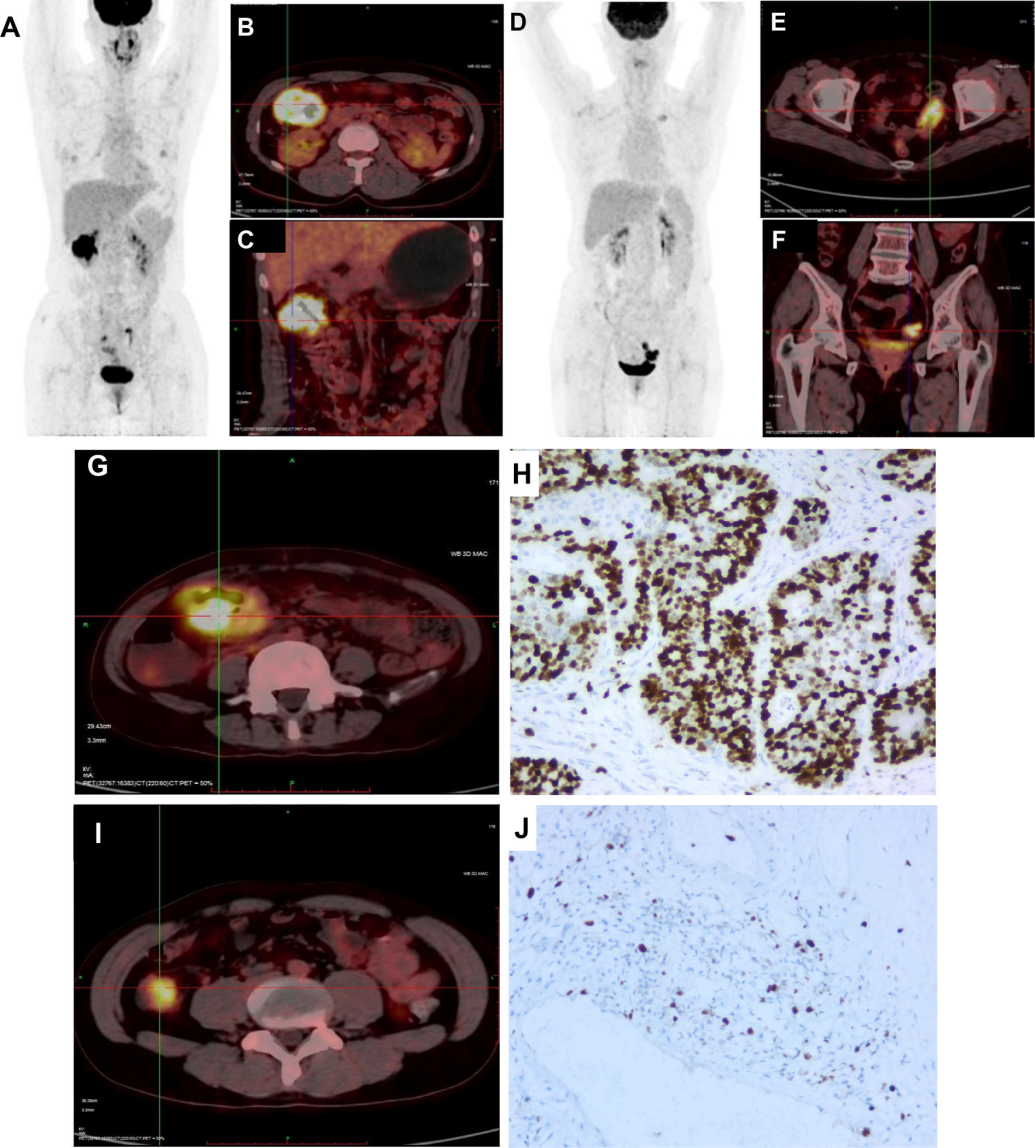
Representative images of KRAS mutation of ^18^FDG-PET/CT scan and Ki-67 index in CRC patients. (A) 18FDG-PET/CT scans image of CRC patient with mutated-type KRAS. (B, C) 18FDG-PET/CT scans showed intense accumulation of 18FDG in the hepatic flexure of colon (SUV: 20.0). (D) 18FDG-PET/CT scans image of CRC patient with wild-type KRAS. (E, F) 18FDG-PET/CT scans showed intense accumulation of 18FDG in the sigmoid colon (SUV: 12.4). (G) 18FDG was accumulated in the sigmoid colon (SUV: 21.3). (H) Maximum IHC expressions are 77.8% for Ki-67. (I) 18FDG was accumulated in the sigmoid colon (SUV: 12.4). (J) Maximum IHC expressions are 22.7% for Ki-67.
